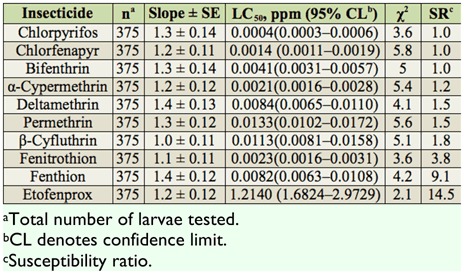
Summary of toxicities of 10 insecticides to third instars of the HS-CT strain of *Culex tritaeniorhynchus* based on the 24 hr exposure contact mortality bioassays.
